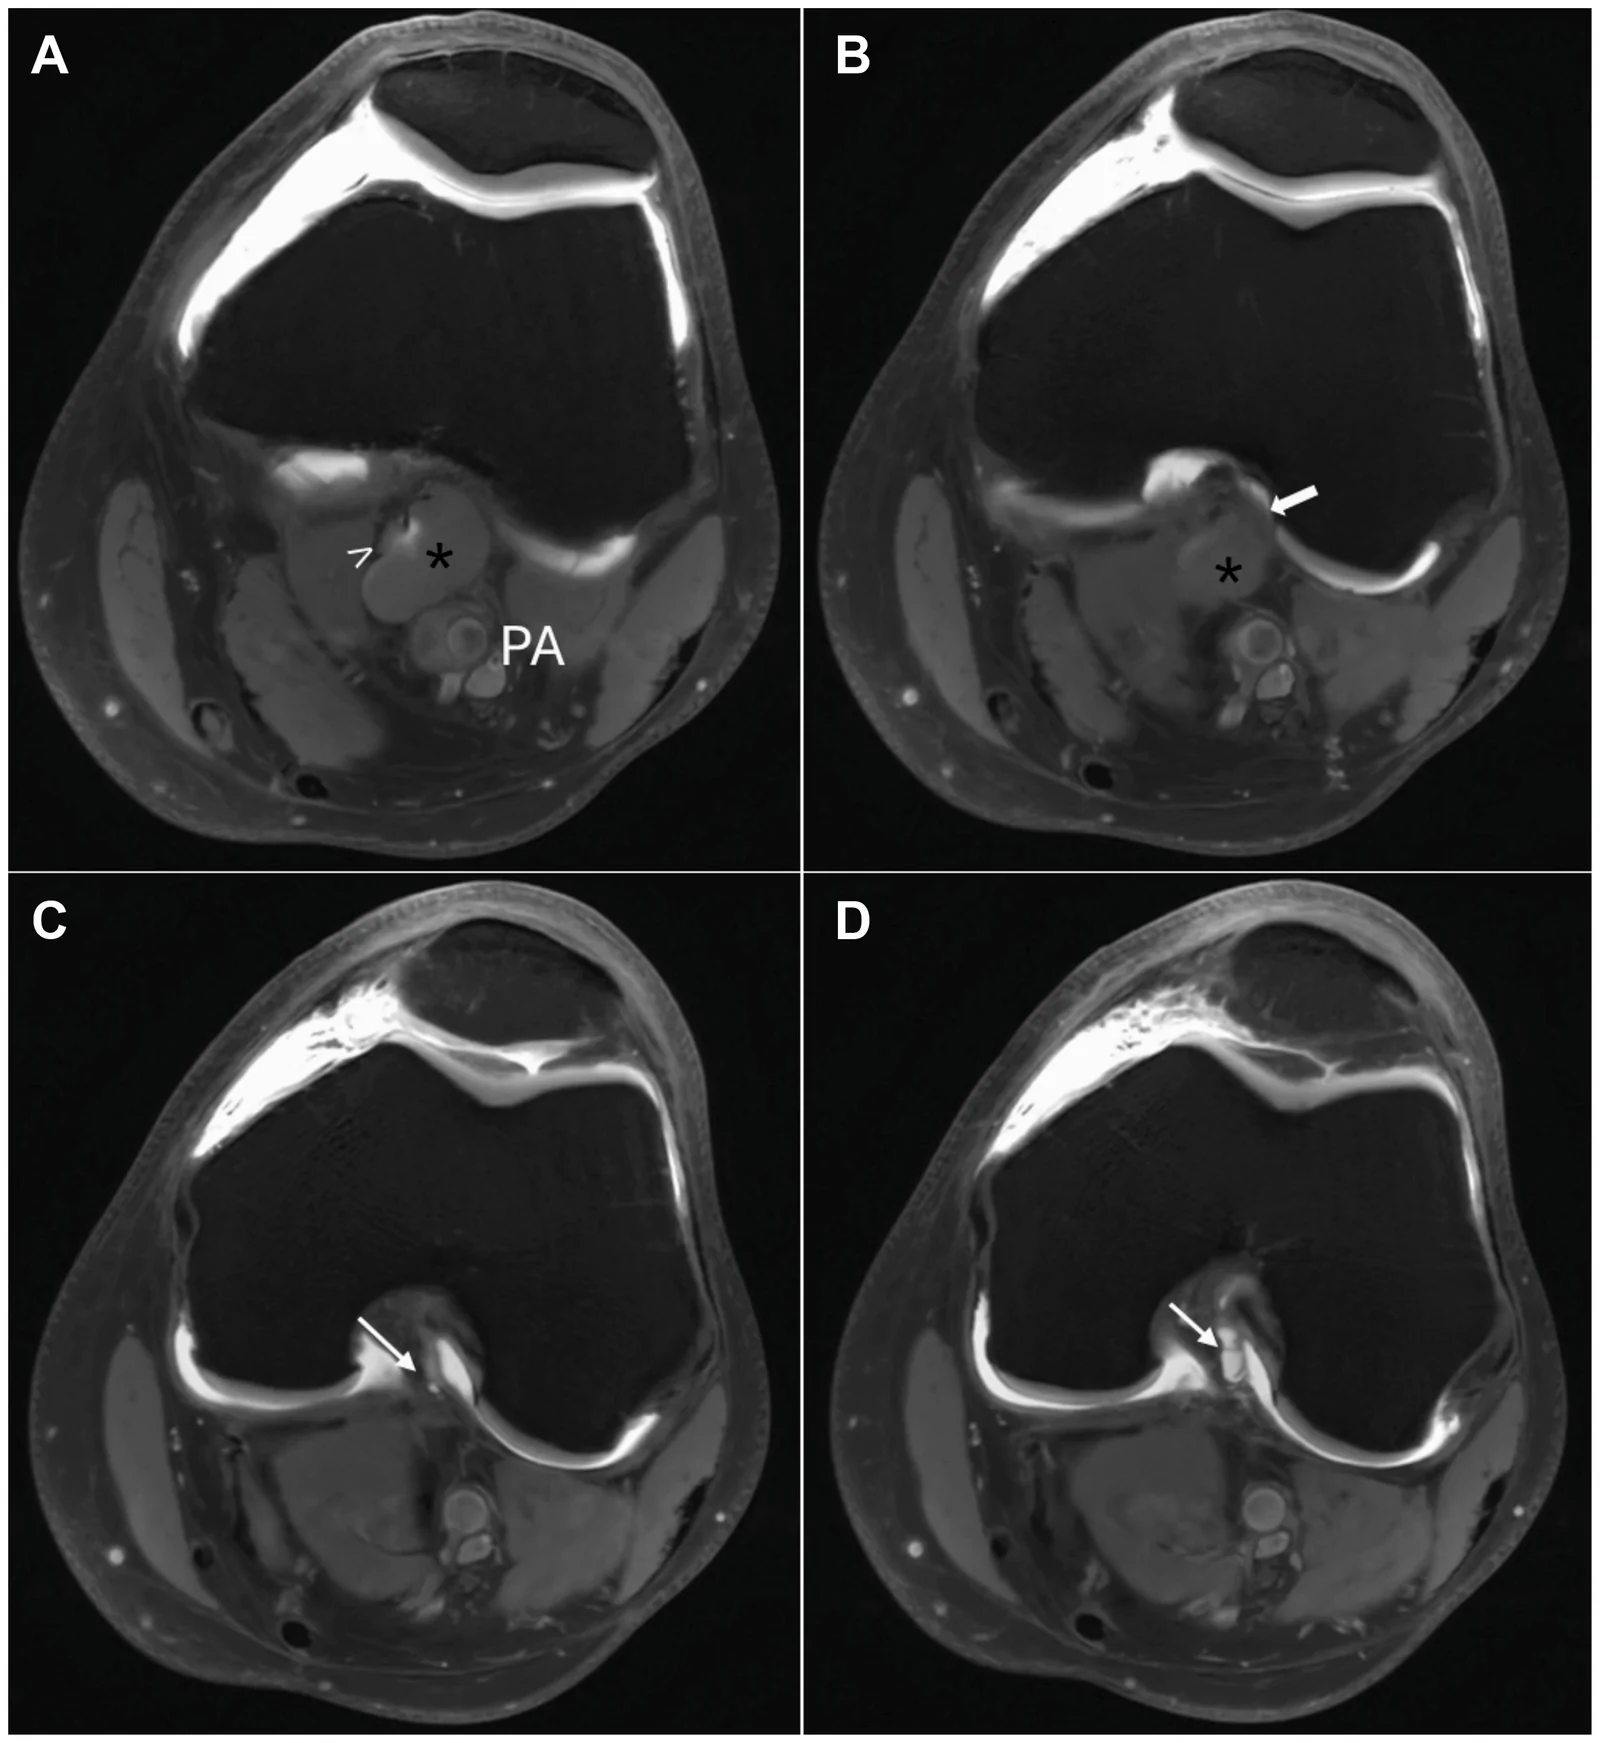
Delayed axial fat-suppressed T1-weighted magnetic resonance (MR) arthrographic images of the left popliteal fossa obtained after knee flexion and extension. A, The adventitial cyst (∗) surrounds the popliteal artery (*PA*), with arthrographic contrast within the cyst (*arrowhead*), confirming communication with the knee joint. B, The communicating neck (*bold arrow*) connects the cyst to the posterior joint capsule. C and D, Consecutive images show the joint-cyst communication (*white arrows*). *∗*, Cyst.
